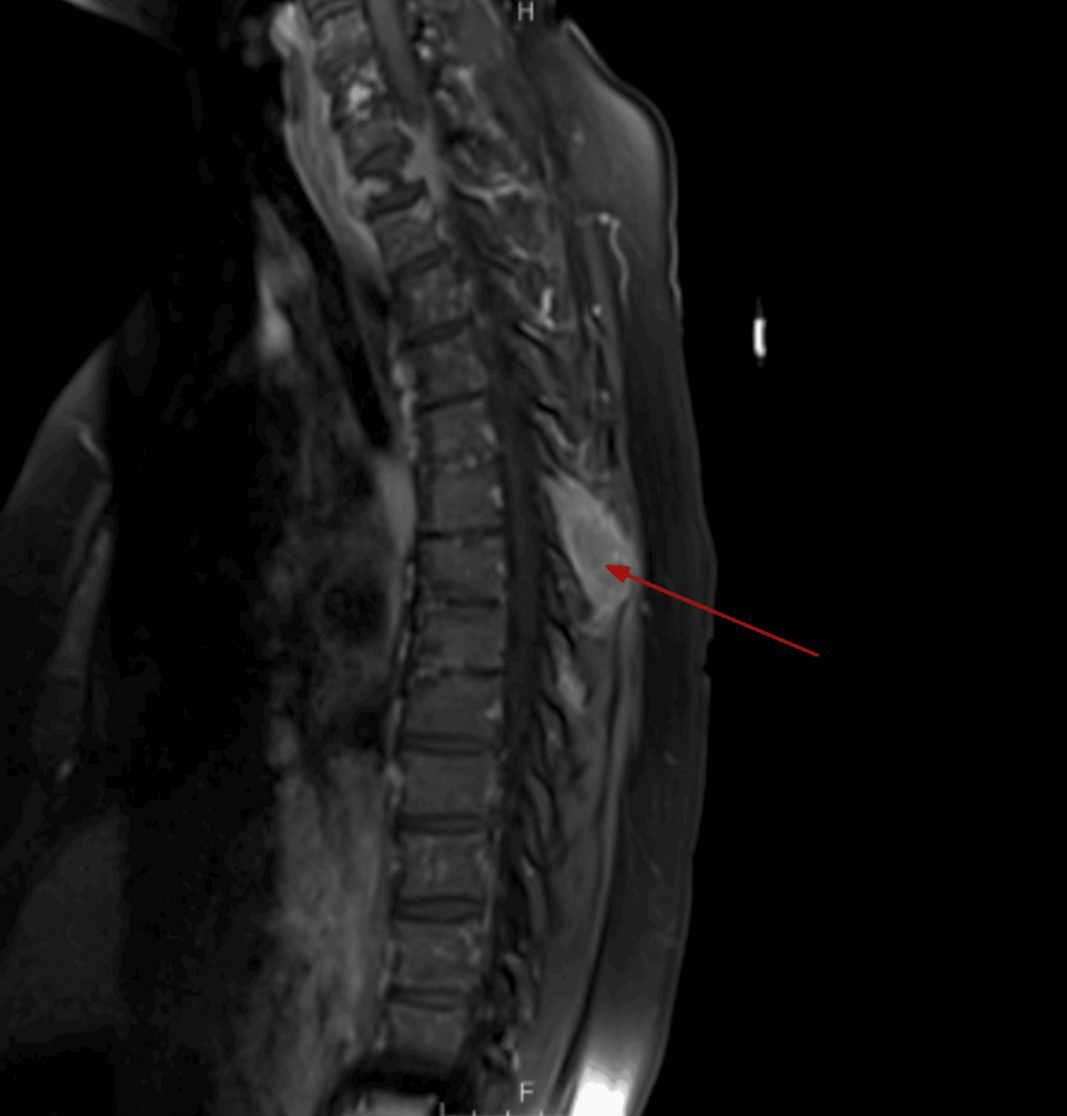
Sagittal T1 MRI showing mild vertebral retropulsion, possible posterior epidural involvement, and moderate spinal canal stenosis
